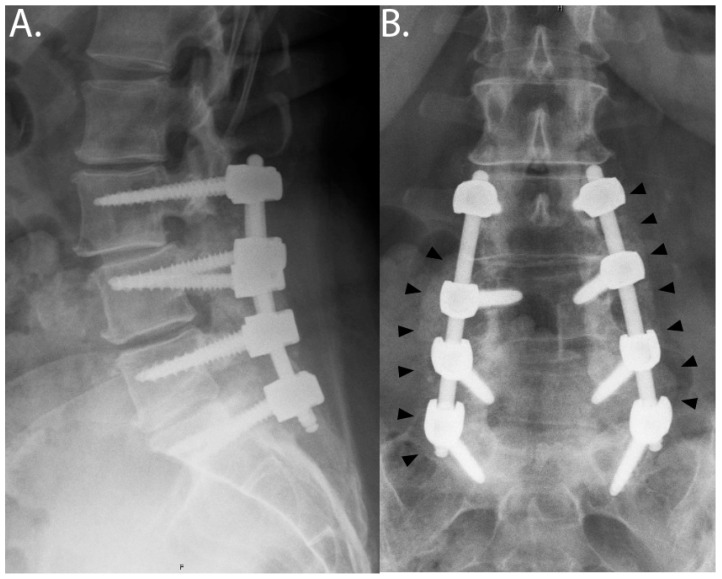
L3-S1 posterior instrumented fusion utilizing HCCP. Bony fusion is annotated with small black arrows. Upright x-rays were taken 13 months post-operatively: (A) lateral, (B) anterior-posterior views.
